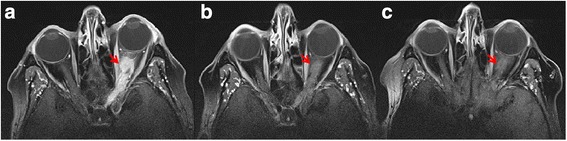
Orbital MRI shows a perineural mass of the left optic nerve (arrows). a A contrast-enhancing lesion compressing and infiltrating the left optic nerve is seen on the initial MRI scan. b A second fat suppression MRI scan shows a perineural T1 hypo-intense mass reaching from the bulbus oculi up to the optic chiasm, with homogeneous contrast enhancement. c Two months after the start with steroids, a distinctly smaller orbital mass is seen on fat suppression MRI scan with residual perineural thickening of the optic nerve without contrast enhancement
